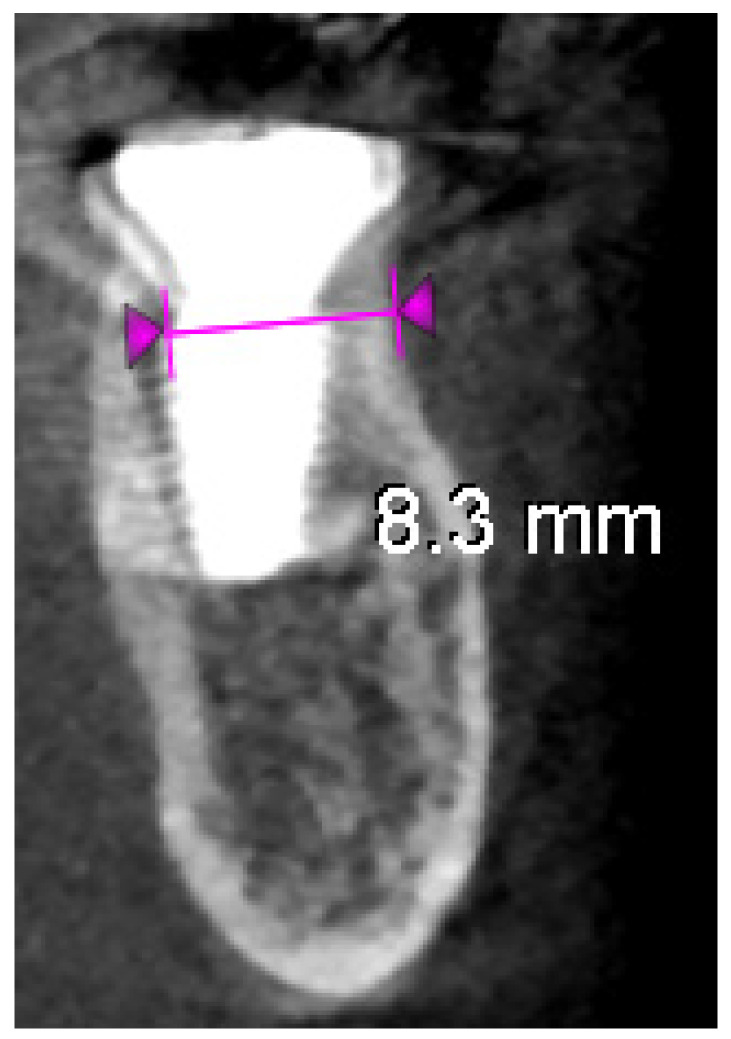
Postoperative CBCT scan showing the implant centrally positioned in the socket.
